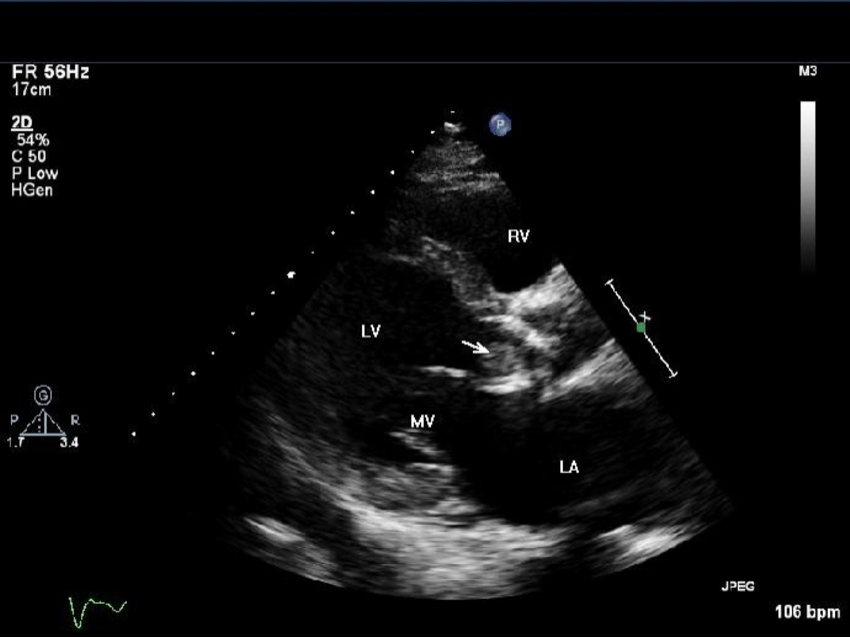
Echocardiogram (parasternal long axis view) showing large vegetation in the tissue prosthetic aortic valve (white arrow). LA = left atrium; LV = left ventricle; MV = mitral valve; RV = right ventricle.
